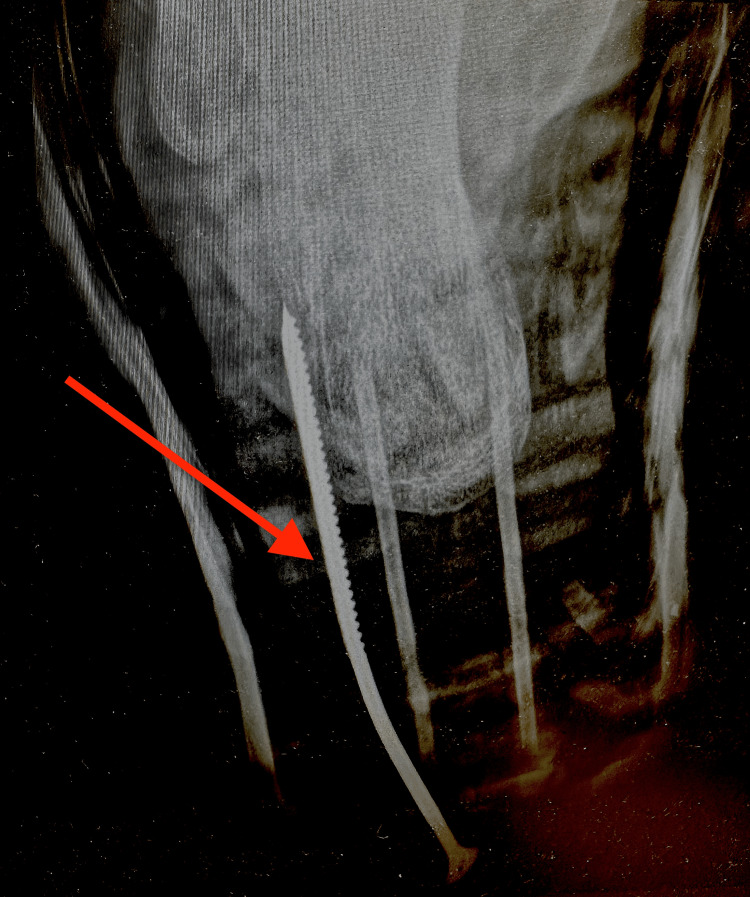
Additional pre-removal radiographic view of the wood screw trajectory. This radiographic projection demonstrates the metallic wood screw within the plantar soft tissues adjacent to the calcaneus, without evidence of bone injury. The red arrow indicates the position of the screw.
